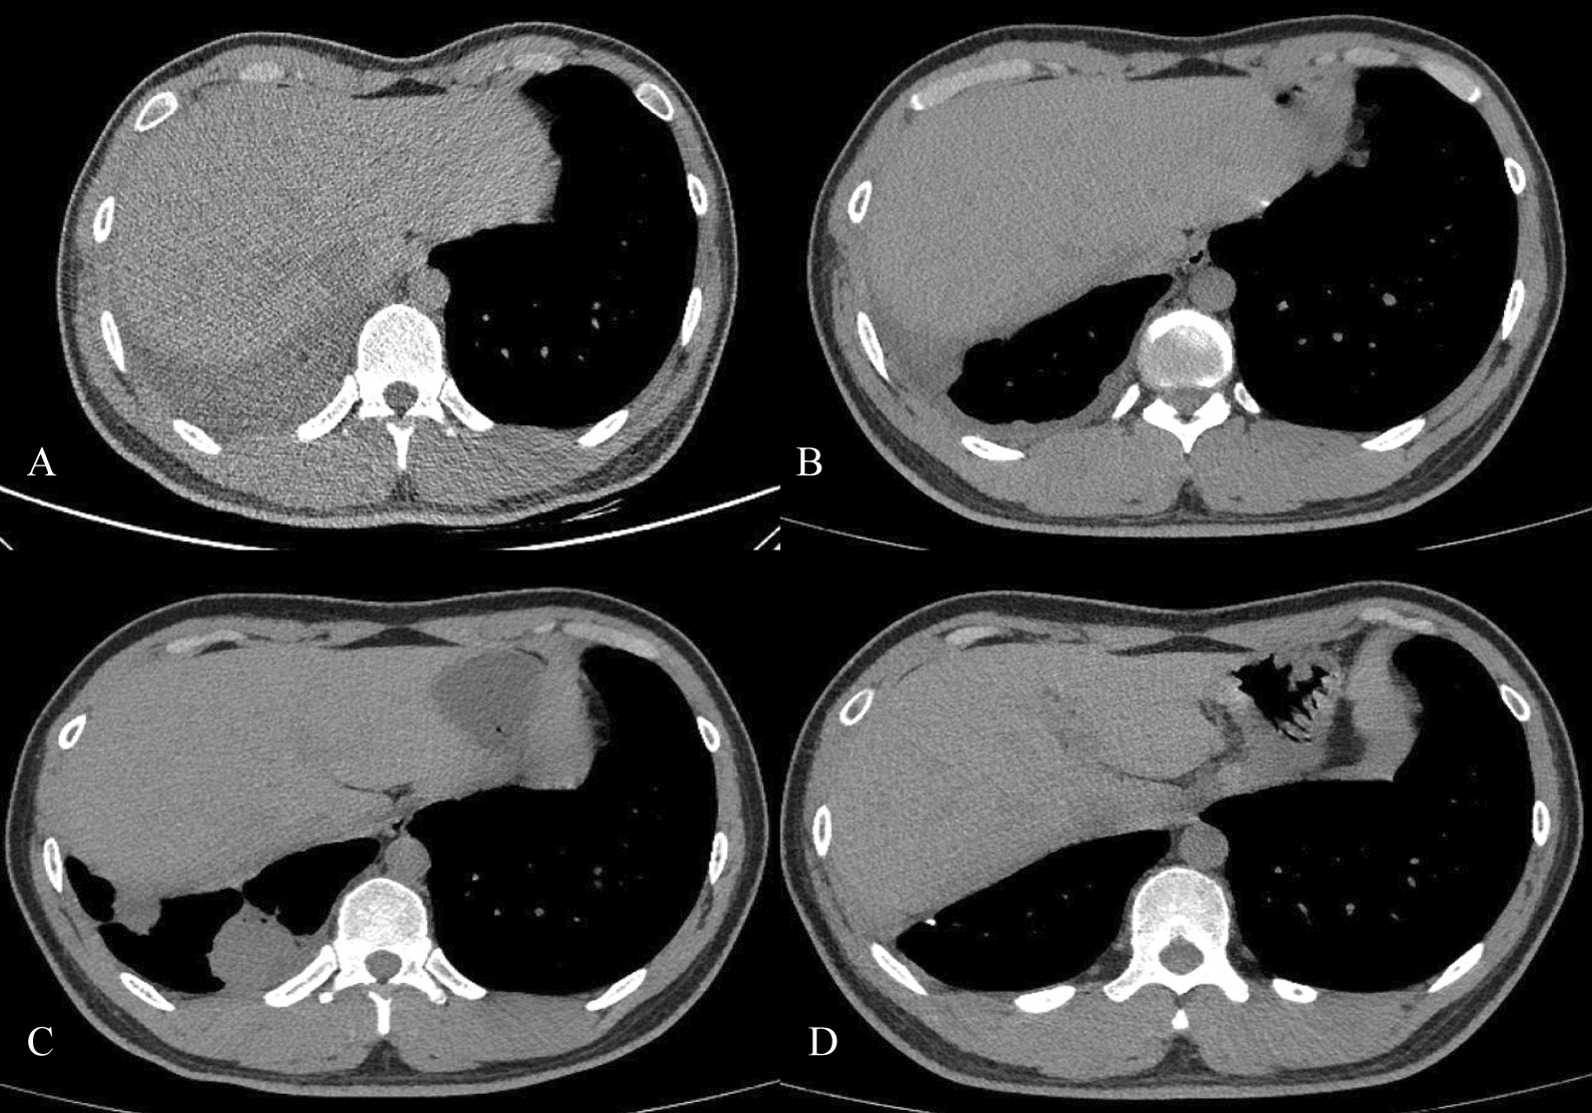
Occurrence and change of pleural-based mass was observed by Chest CT. A shows the large amount of pleural effusion in the right chest; B shows that pleural effusion was unapparent after draining the pleural fluid and starting the treatment; C shows the occurrence of a pleural-based mass in the same site of the right chest after four months of chemotherapy; D shows a pleural-based mass resected by surgery, with no recurrence in a year's follow-up
